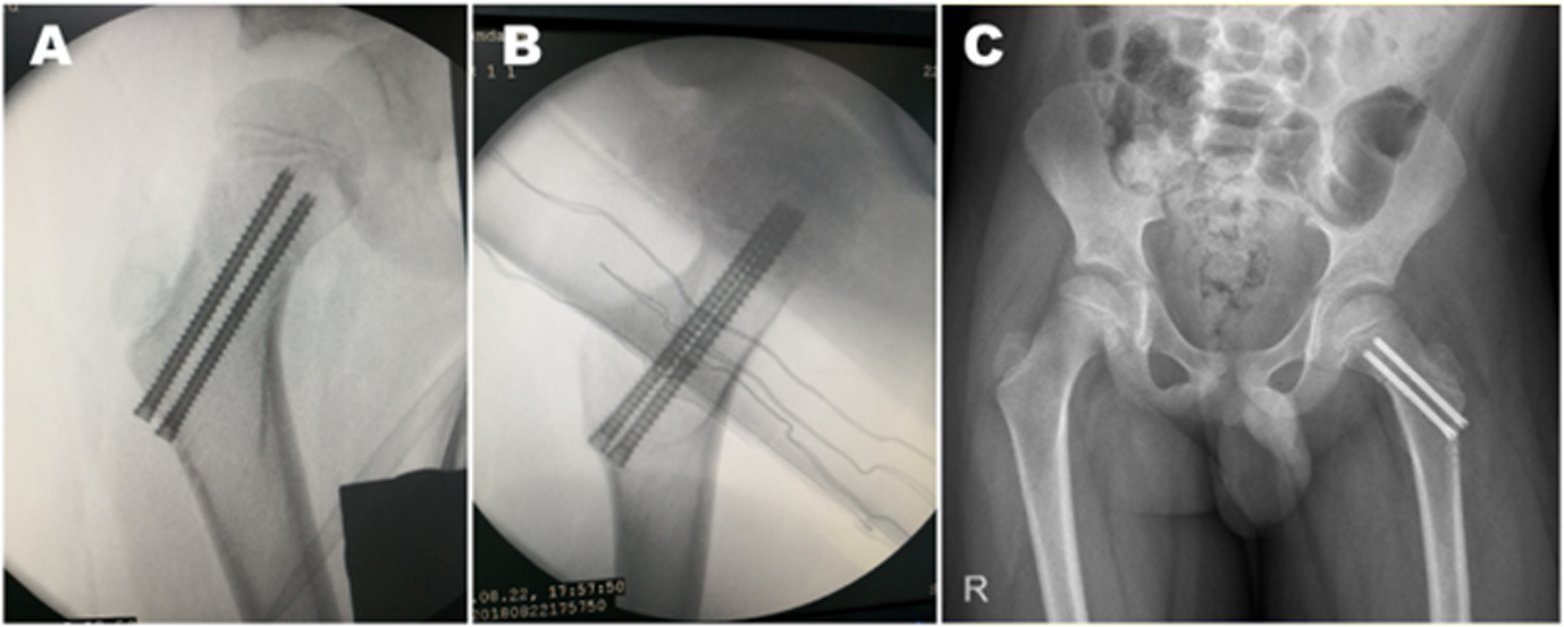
X-ray radiography during the surgery. (A–C) Two HCCSs were inserted after closed reduction of the fracture, and the extent of fracture reduction was evaluated based on anteroposterior and lateral x-ray radiographs obtained during the surgery (A,B) and anteroposterior radiographs obtained on the day after surgery (C).
